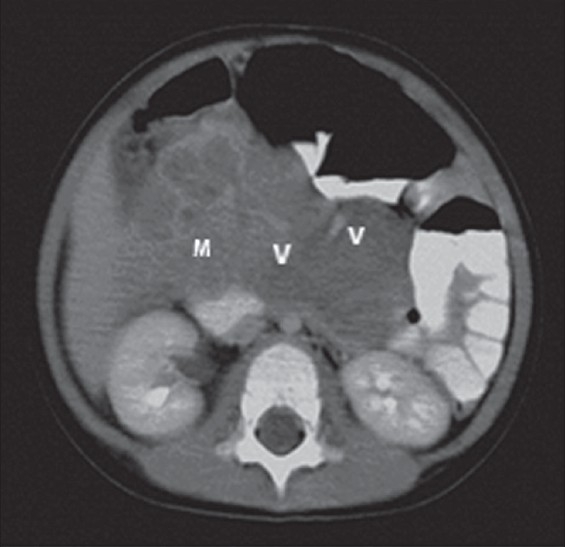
The first contrast-enhanced computed tomography (CECT) scan of the abdomen shows the heterogeneously enhancing mass (M) anterior to the aorta and inferior vena cava. The superior mesenteric vessels (V) are displaced anteriorly
